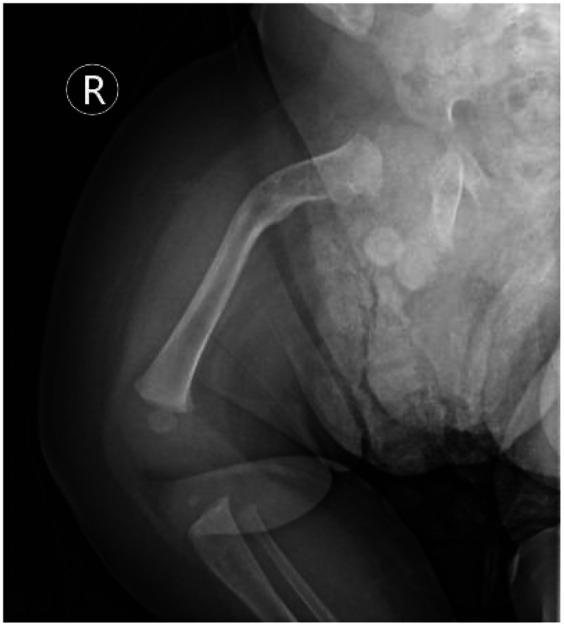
Radiographic outcome at the final follow-up (day 136 of life). Final follow-up radiograph of the right femur demonstrates complete bony union with remodeling and consolidation of the previously formed callus (red arrow). Despite fracture healing, the underlying bony deformity and bowing of the femur persist. This image shows the final outcome of the fracture and underscores the persistent nature of the skeletal abnormalities in OI, which coexist with the fracture healing process.
